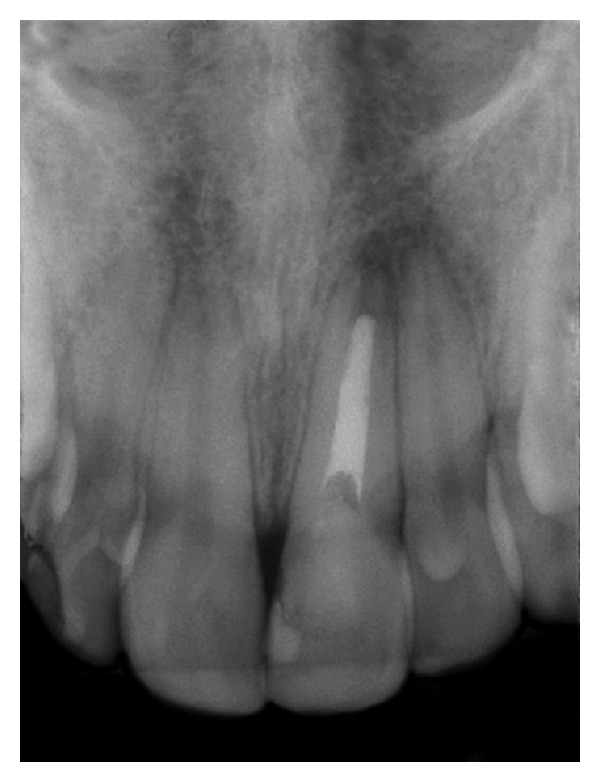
Radiographic view of the fractured tooth.
